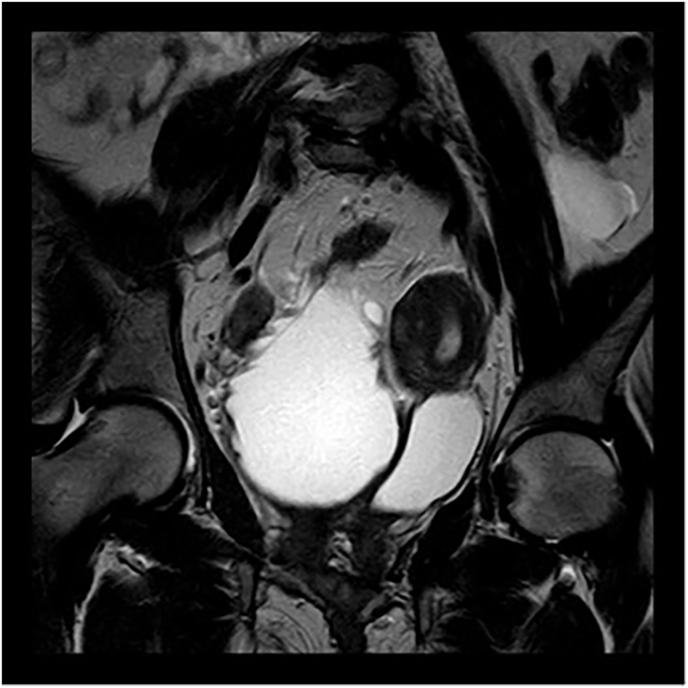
Magnetic resonance imaging, coronal plane. Duplicated bladder.
